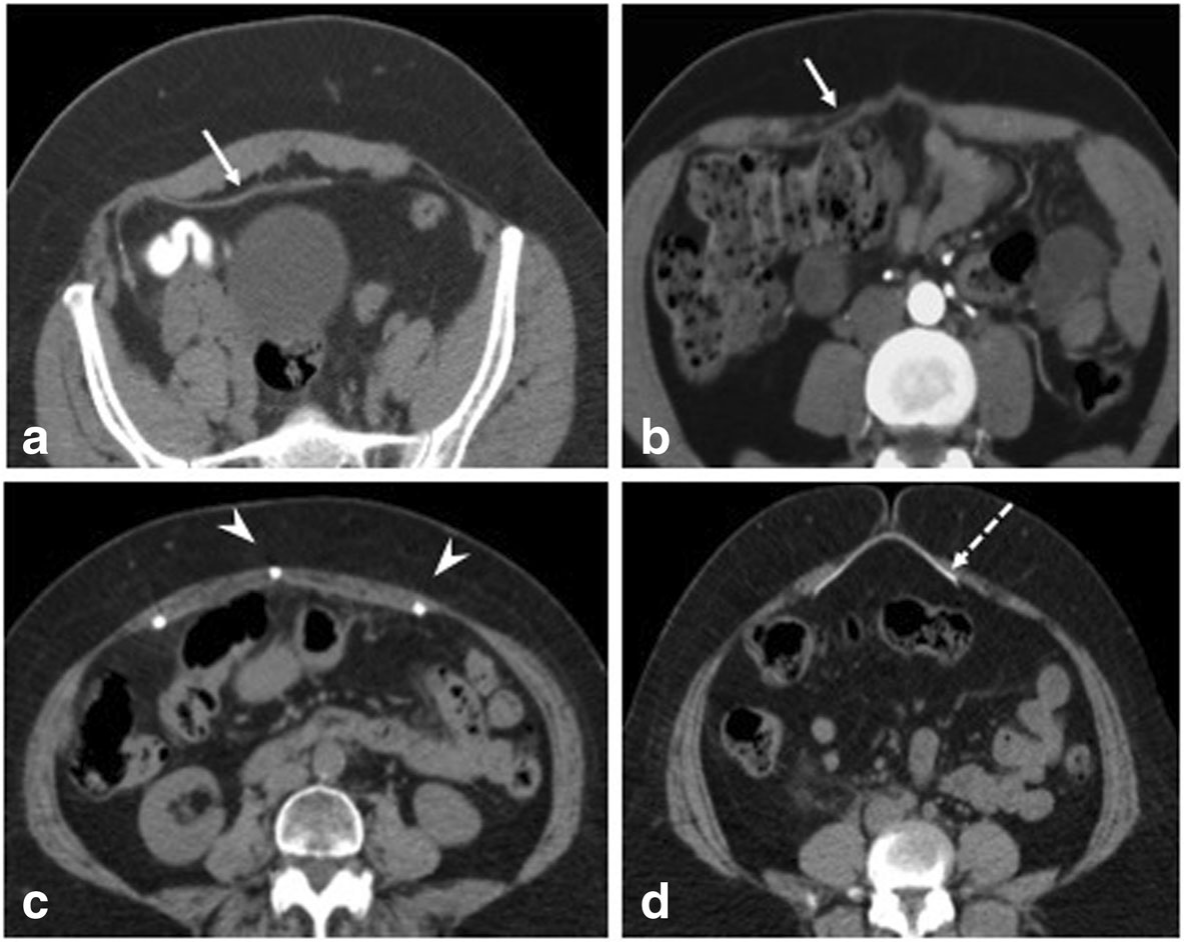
Mesh appearances on CT axial sections. a Preperitoneal placement of Prolene-based mesh appearing isodense to muscle. Presence of fat can aid in better visualization of the mesh. b Inlay Prolene-based mesh placement (arrow). c Laparoscopic intraperitoneal placement of mesh with tackers (arrowheads). d Mesh made of PTFE appearing radiodense (dashed arrow)
